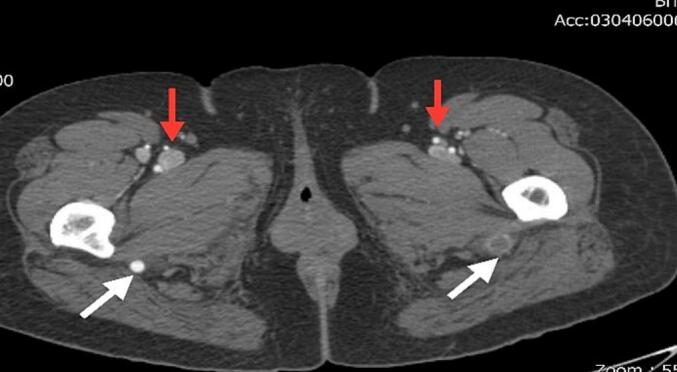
Axial CT angiogram demonstrated bilateral persistent sciatic arteries (white arrows) with aneurysmal dilatation left sciatic artery and complete thrombosis proximal part, femoral arteries (red arrows) are hypoplastic.
